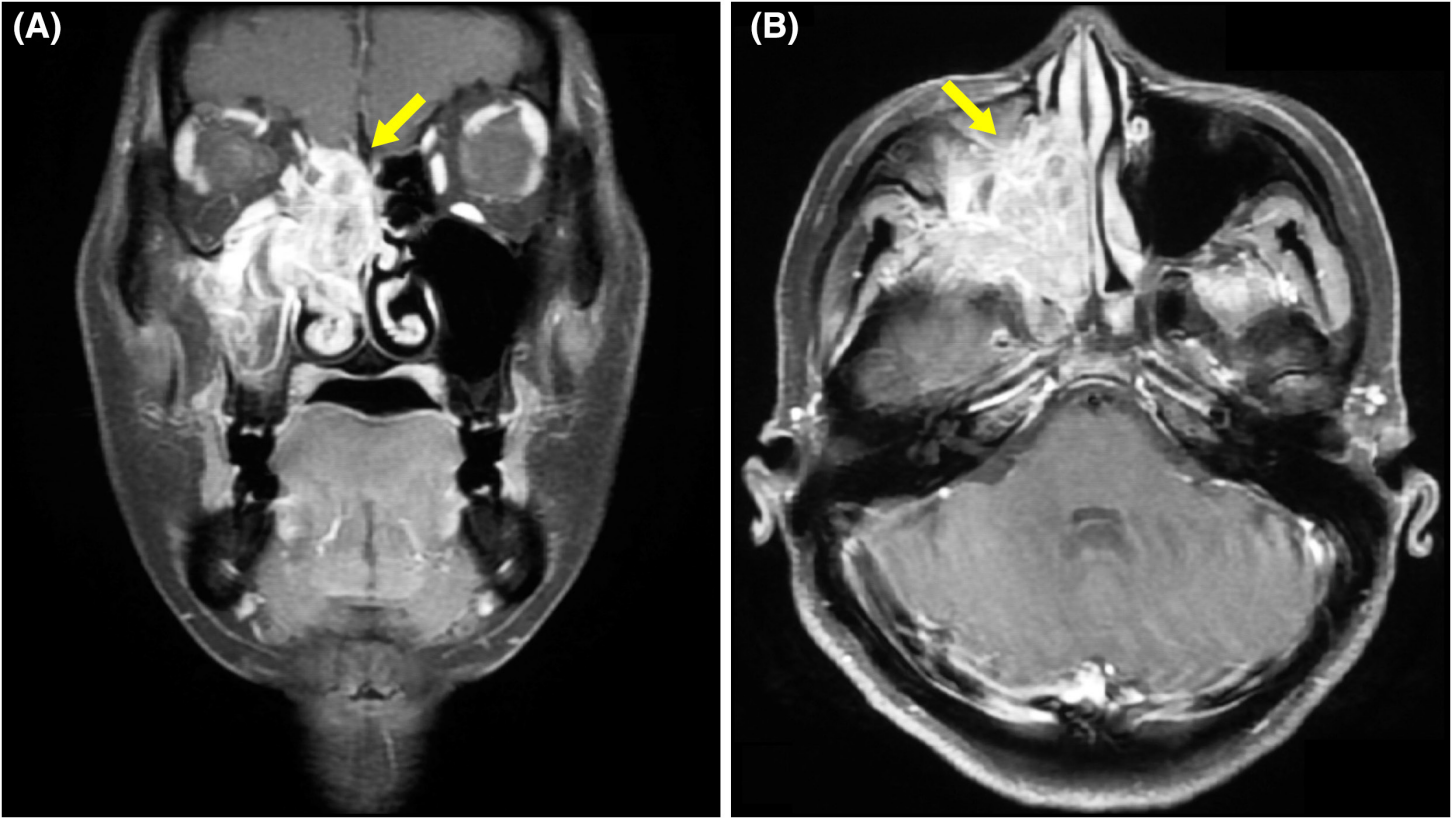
Coronal (A) and axial (B) magnetic resonance imaging of the head revealing a large homogenous well‐enhanced mass (42 × 21 mm) in the right ethmoid sinus (yellow arrow). The mass had extended to the right sphenoid sinus, nasal cavity, and orbit
